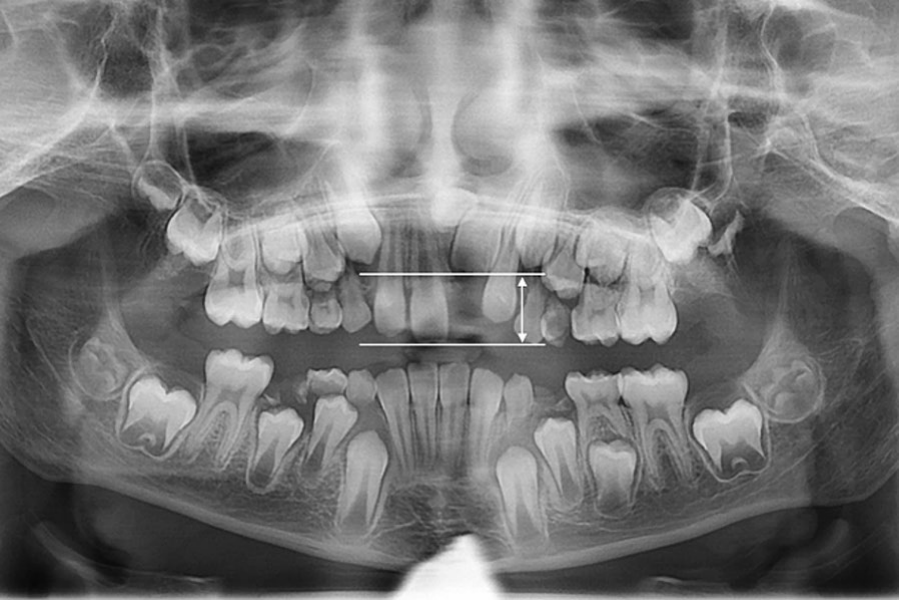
Panoramic X-ray measurement of the degree of impaction from the incisal edge of the impacted tooth to the incisal edge of the neighboring tooth
